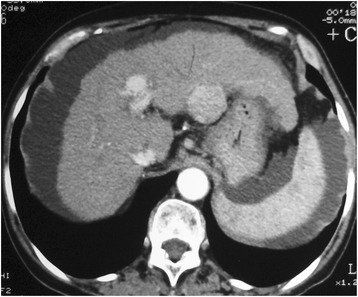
Computed tomography before DEB TACE. Computed tomography showing a hypervascular liver tumor in the left lobe compatible with Hepatocellular Carcinoma in a 71 year-old female patient with liver cirrhosis and hepatitis C
